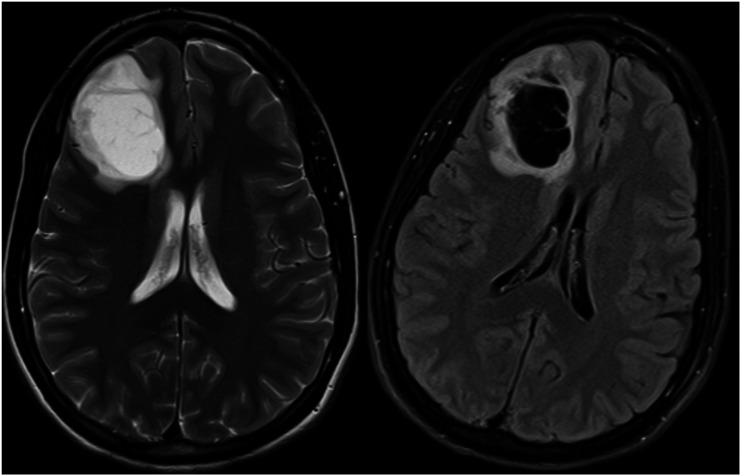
IDH-mutant, 1p/19q non-codeleted glioma in a 25-year-old male. The tumor harbors a large cystic component that follows the cerebrospinal fluid signal on the T2WI (left) and FLAIR (right), which does not qualify as T2FMM.
